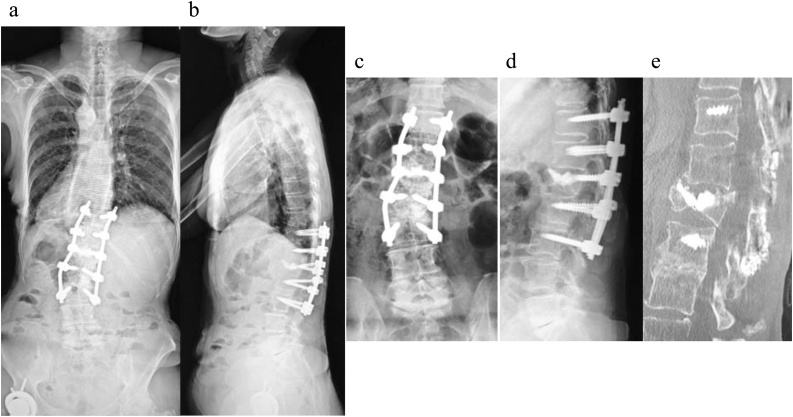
Postoperative radiographs and CT images at 1-year postoperatively. One-year postoperative radiographs: (a) Standing whole spinopelvic AP radiograph, (b) Standing whole-spinopelvic lateral radiograph, (c) Lumbar AP radiograph, (d) Lumbar lateral radiograph. (e) Sagittal image reconstruction of CT images at 1-year postoperatively.
